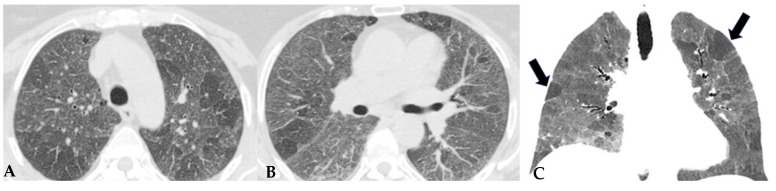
NFHP: chest HRCT ((A,B): axial scan; C: MPR coronal). GGO pattern and faint centrilobular nodules are observed in both upper lobes and apical segments of the lower lobes (A,B). Areas of mosaic attenuation (black arrows) are better defined in the coronal MPR reconstruction (C). The pattern is suggestive of an acute, non-fibrotic expression of hypersensitivity pneumonitis (NFHP).
